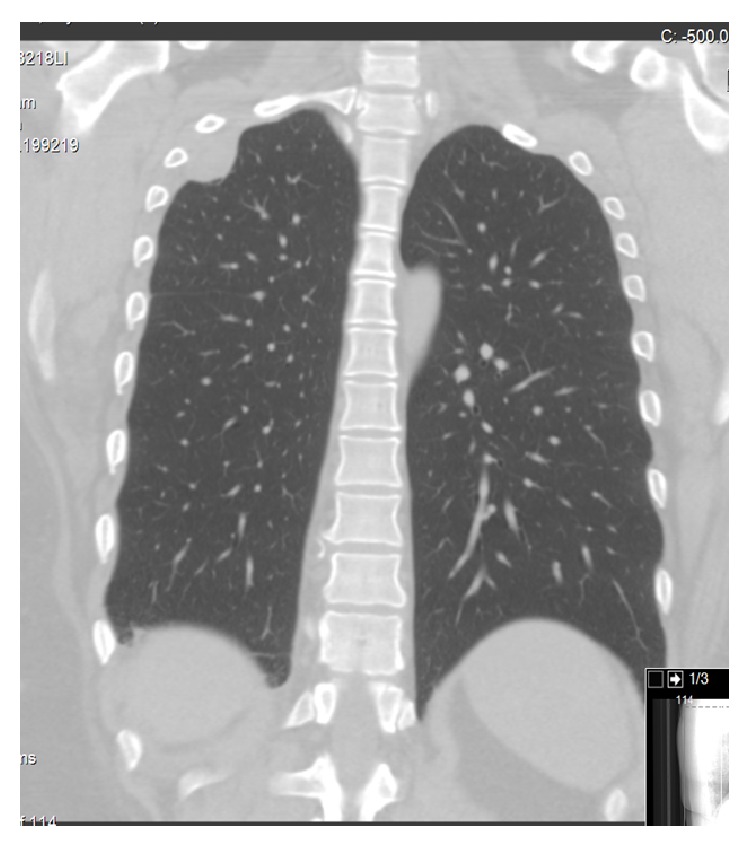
Chest CT after treatment.
